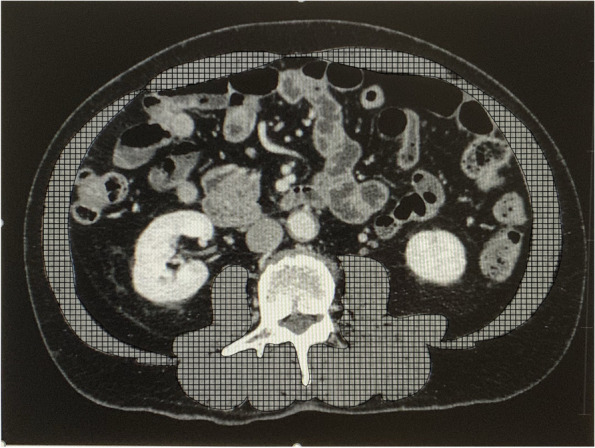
Evaluation of skeletal muscle mass volume. Axial computed tomography image of the abdomen at the third lumbar vertebra. The skeletal muscle area is highlighted in hatching (Hounsfield unit threshold from − 29 to + 150)
